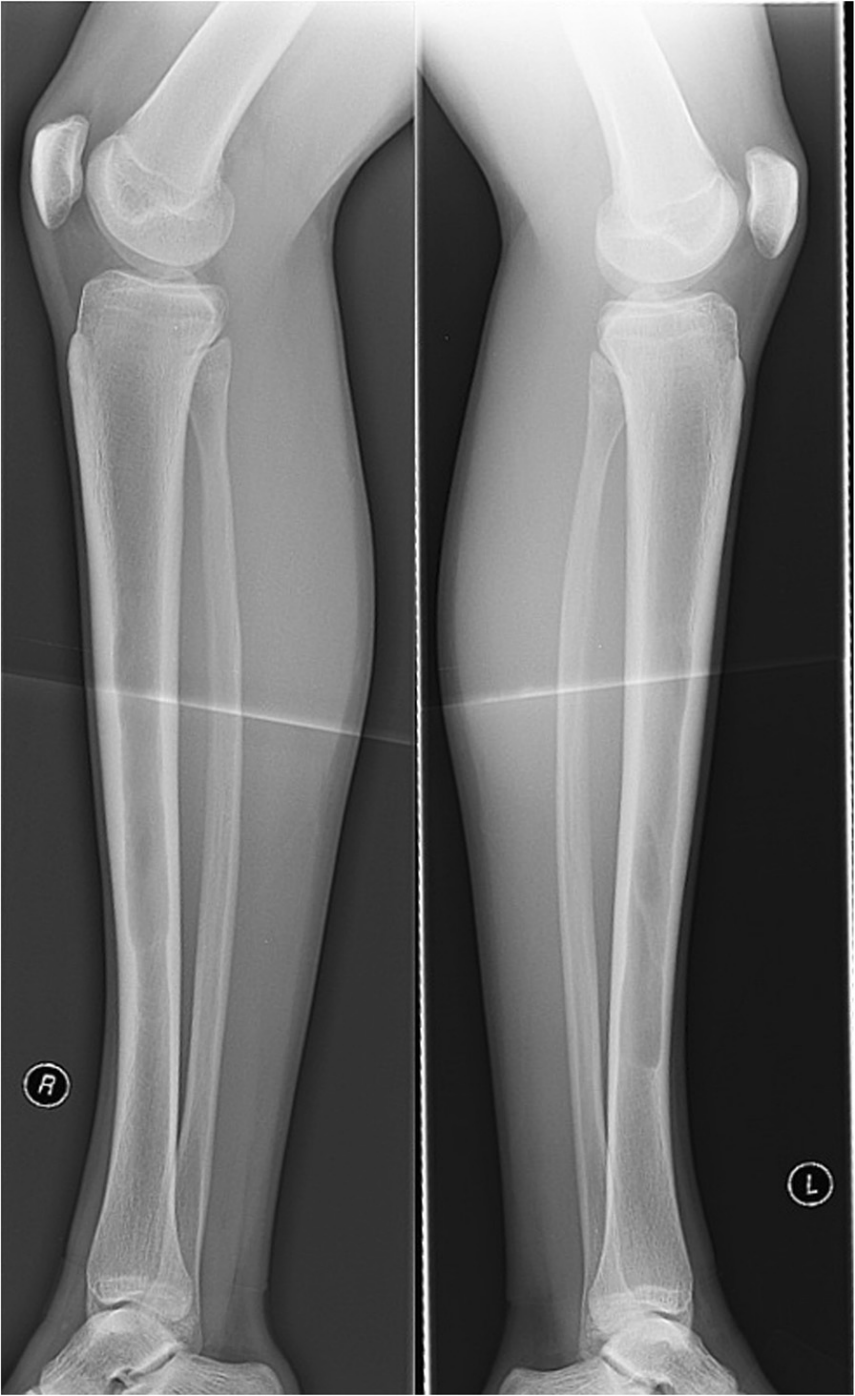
Lateral x-rays. Similar to the a.p. view, these findings would have been easy to misinterpret or miss without additional MRI
